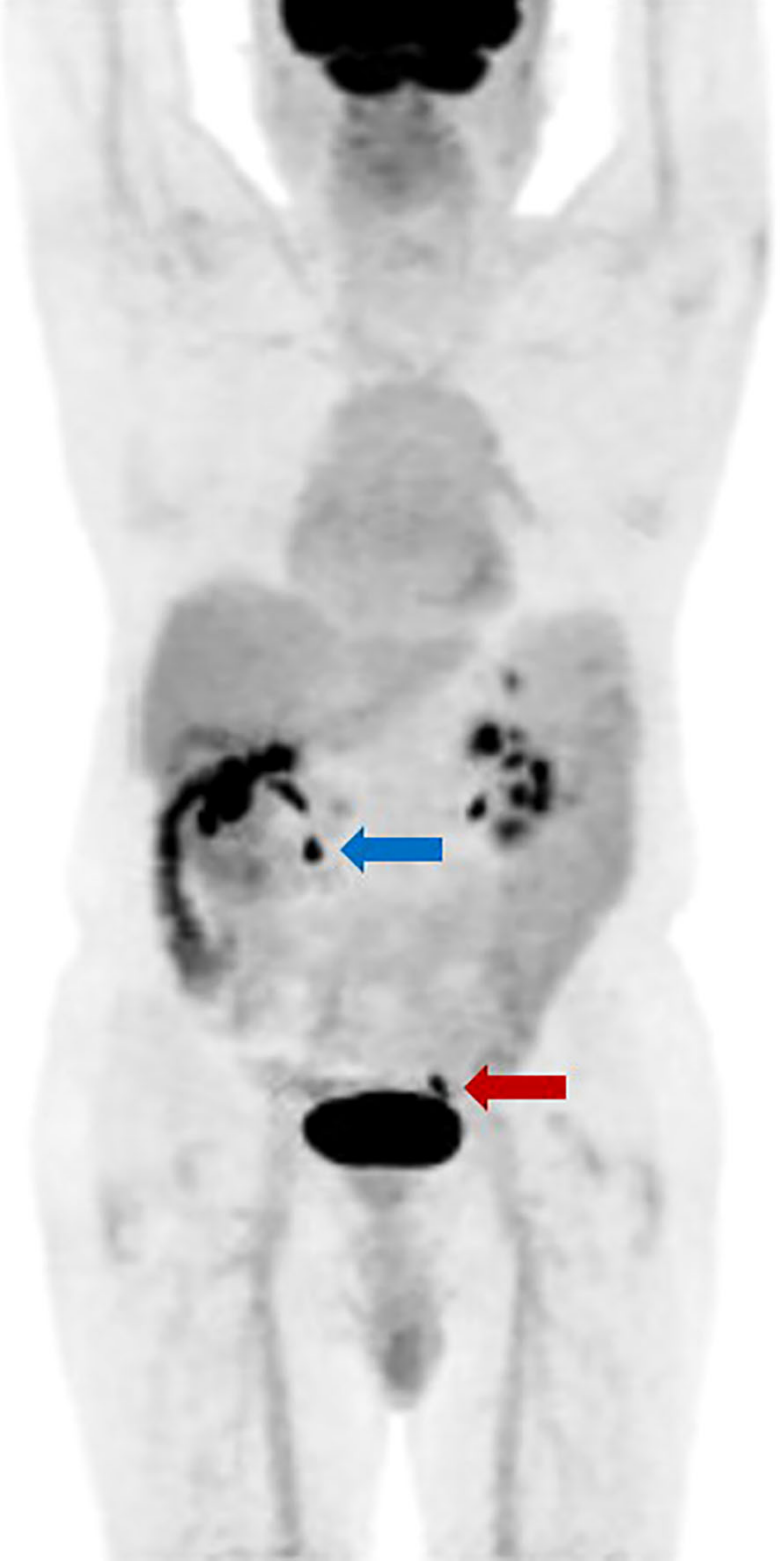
PET/CT scanning showing increased metabolic activity in the prostate. The patient exhibits slightly increased metabolic activity in the posterior prostate, with calcification spots noted (indicated by a red arrow). The ascending colon shows likely physiological uptake. There is localized increased metabolic activity in the transverse colon (indicated by a blue arrow), suggesting either physiological uptake or the presence of a small adenoma.
